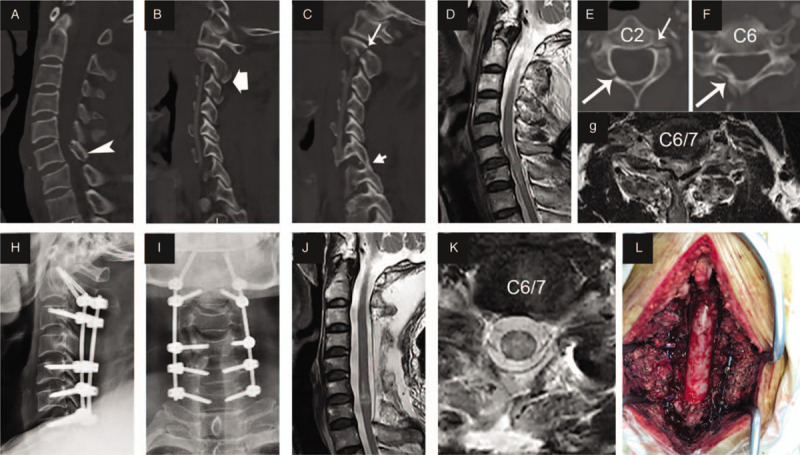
Representational preoperative (A–G), intraoperative (L) and postoperative (H–K) images of a 50-year-old male patient who sustained an atypical hangman fracture, C type fracture of C6 and spinal cord injury. His ASIA motor score (AMS) was 15 and AIS grade A. Sagittal subaxial CT indicated C6 translation rotation injury (A, arrowhead) and atypical hangman fracture (C and E, short arrow) accompanied by fracture of the right inferior articular process of C2 and C3 (B), locked facet of C6–7 (C, arrow), and right lamina fracture of C2 and C6 (E and F, long arrow). MRI (D and G) showed SCI at the C6 to C7 level, and the subarachnoid space (SAS) was absent at C6/7 (G). Laminectomy of C3–7 (L) and pedicle screw fixation from C2 to C7 were performed in this patient 10 h after trauma. Postoperative X-rays (H and I) showed good screw positioning and cervical sequence. MRI indicated successful decompression and opened SAS (J and K) 9 days after trauma. Fifteen days after injury, the patient recovered paralysis with an AMS of 29 and AIS grade B. The patient had mild hyponatremia and no hypotension or tracheotomy during hospitalization.
